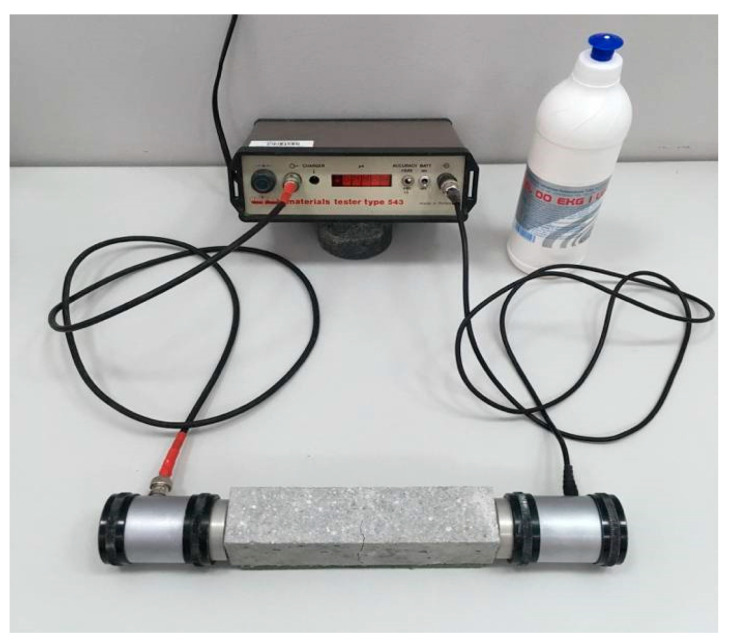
Test stand for testing the ultrasonic pulse velocity by direct transmission in rectangular mortar samples.
